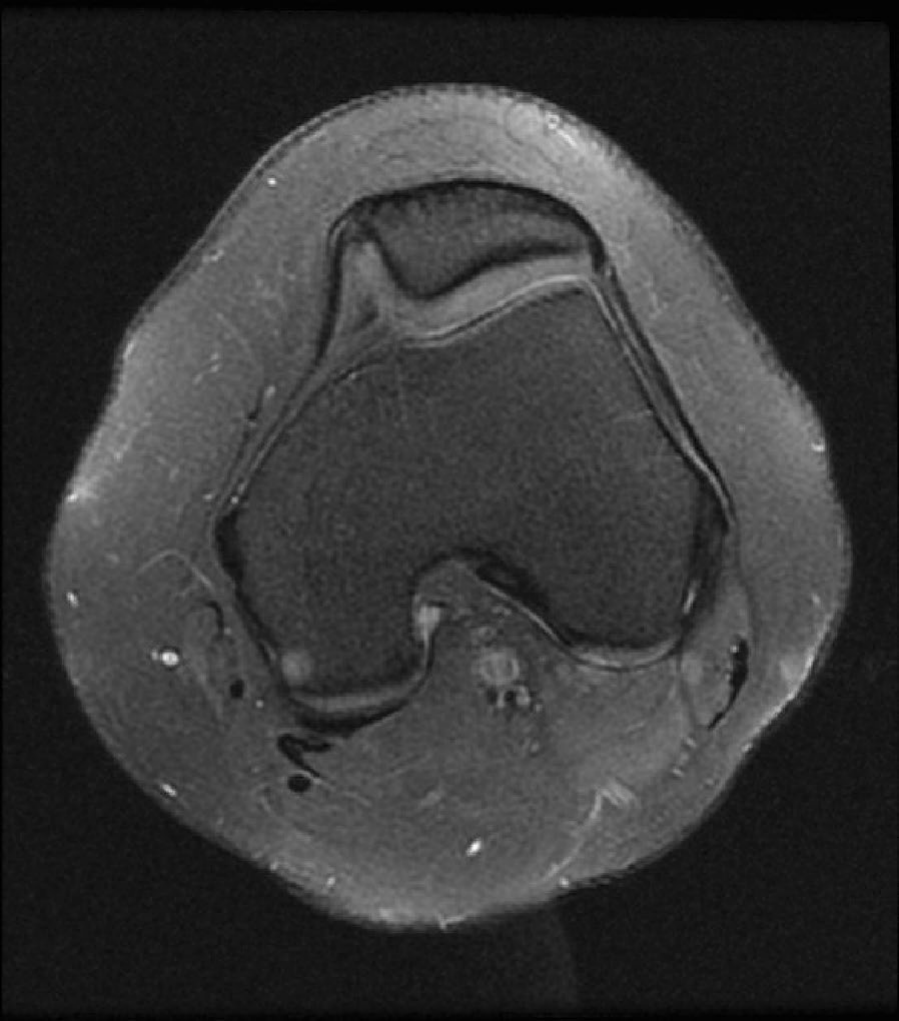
Axial PD-fatsat MRI (a Sect. 3 cm above the knee joint). Type C trochlear dysplasia is present. Note the trochlear fascial asymmetry, increased lateral convection, and medial facet hypoplasia
